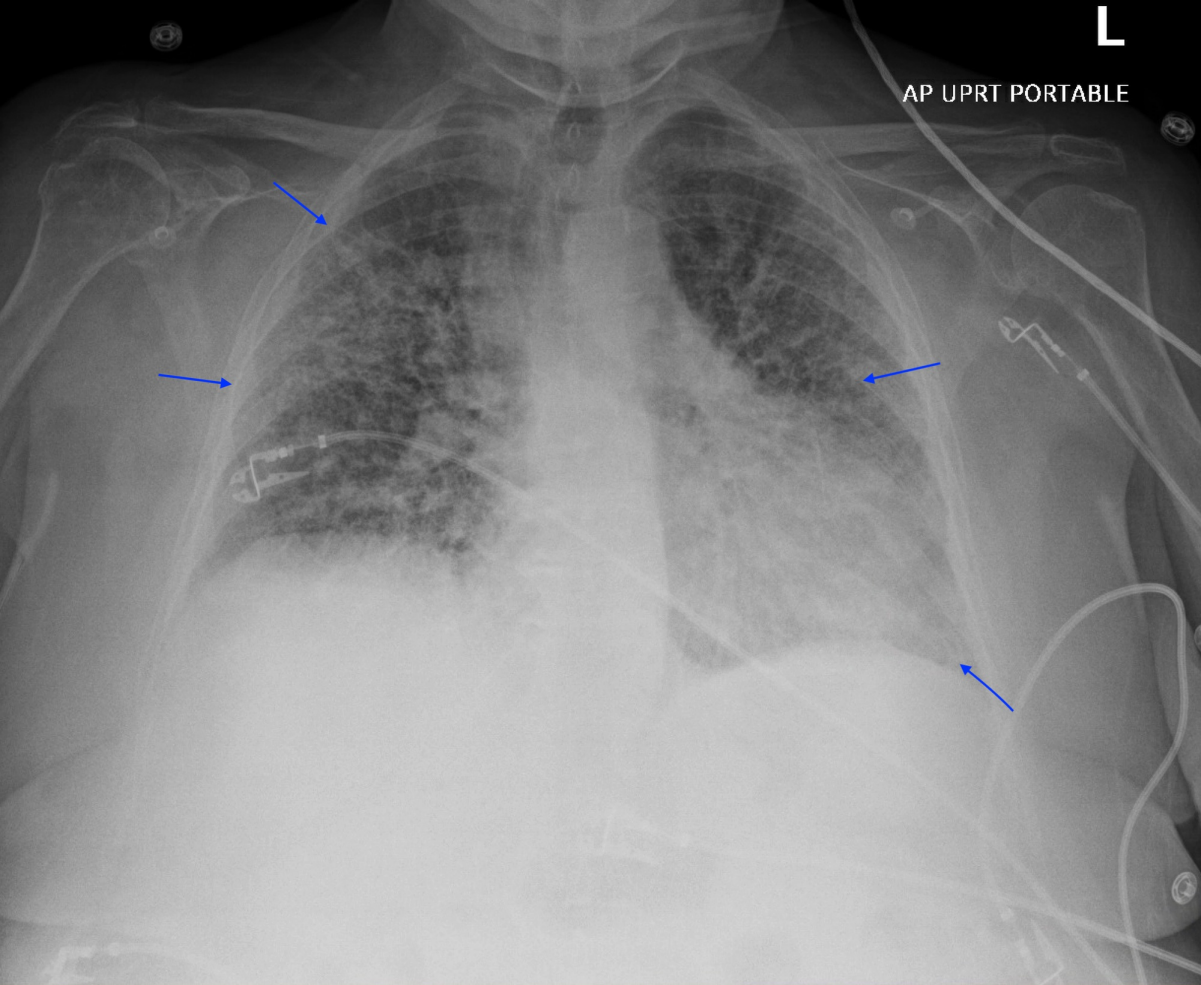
Chest X-Ray Worsening of the bilateral multifocal airspace opacities comparing to prior studies. L: left; AP: anterior-posterior; UPRT: up-right.
